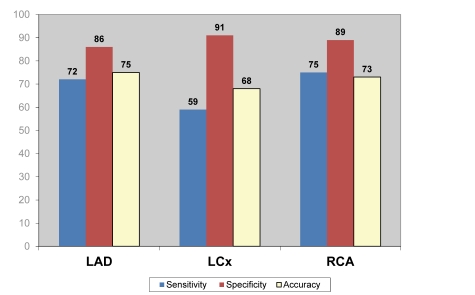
Pooled diagnostic value of SPECT for detection of coronary artery disease based on individual coronary artery assessment.
